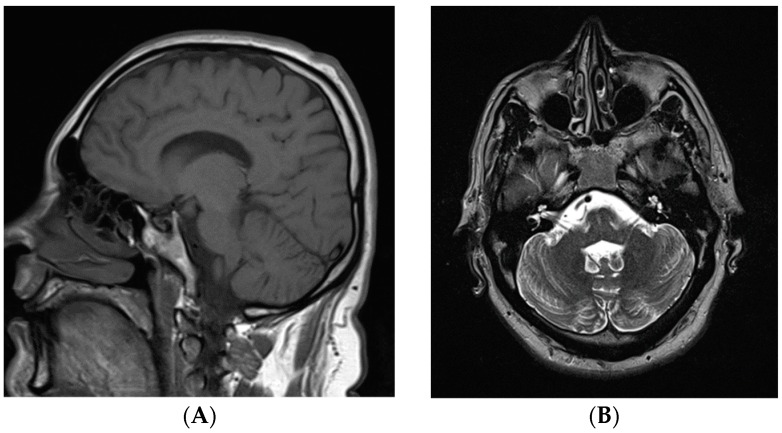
Magnetic resonance imaging of the brain. (A) T1 weighted sagittal view; and (B) T2 weighted axial view. Both images demonstrate the normal appearance of the cerebellum.
